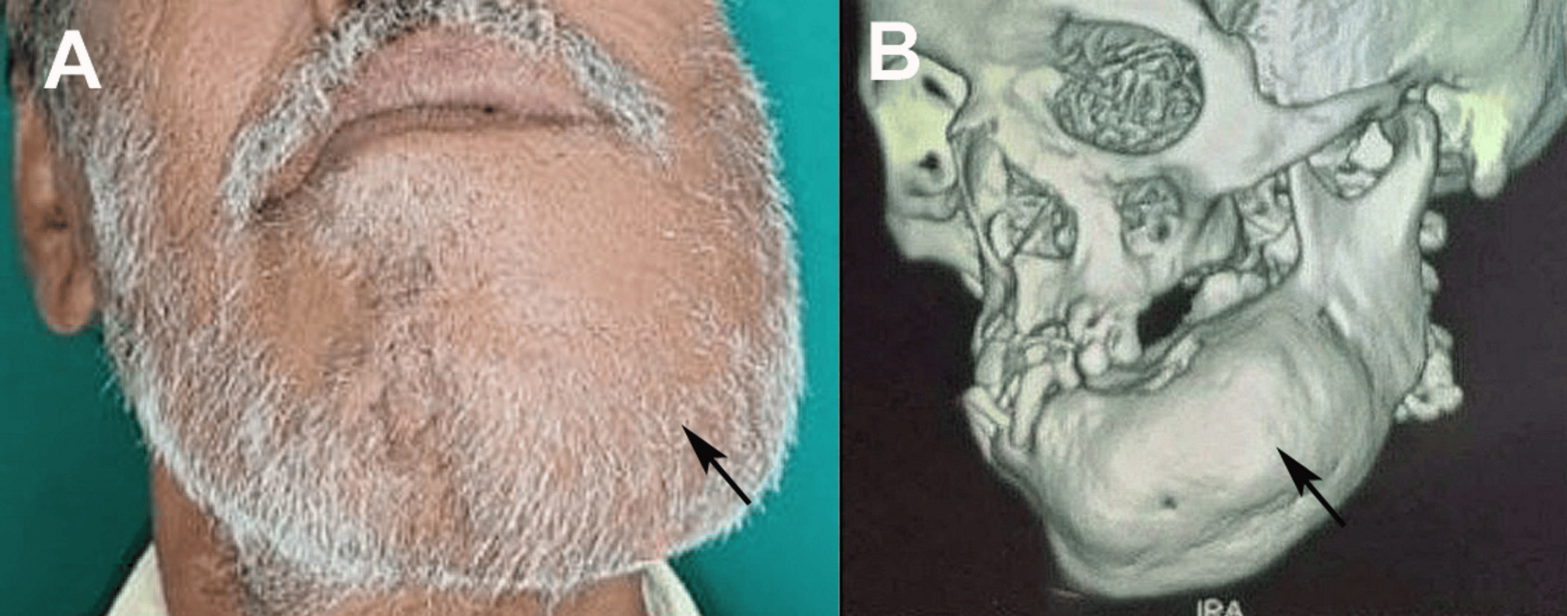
(A) Extraoral swelling on the left side with marked facial asymmetry and (B) CT showing expansion of mandibular bone without perforation on the left side Original images of the patient were used with the patient's consent for publication. CT: computed tomography
